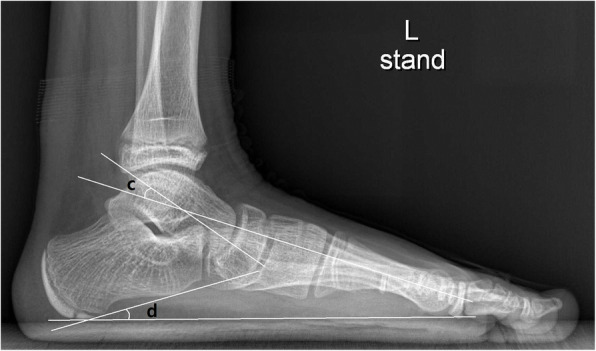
On weight-bearing lateral (LAT) foot radiographs, LAT talo-1st metatarsal angle (c) and calcaneal pitch angle (d) were measured
